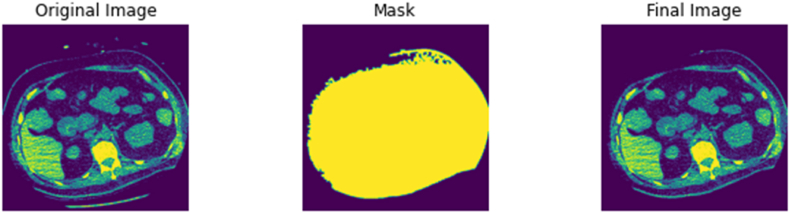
Noise removal and segmentation script on CT scan from the TCIA.
